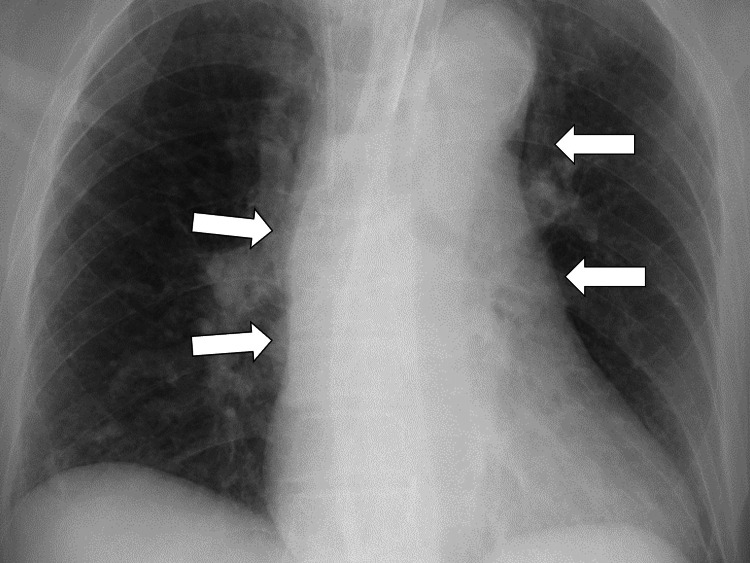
Chest radiography not revealing any mediastinal widening, pleural cap, and no displaced calcium sign (white arrows).
